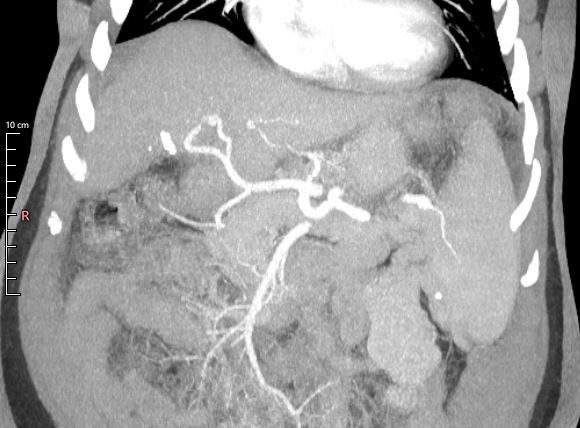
Abdominal computed tomography image with maximum intensity projection reconstructed in the arterial phase, coronal plane, showing an accessory left hepatic artery originating from the left gastric artery.
